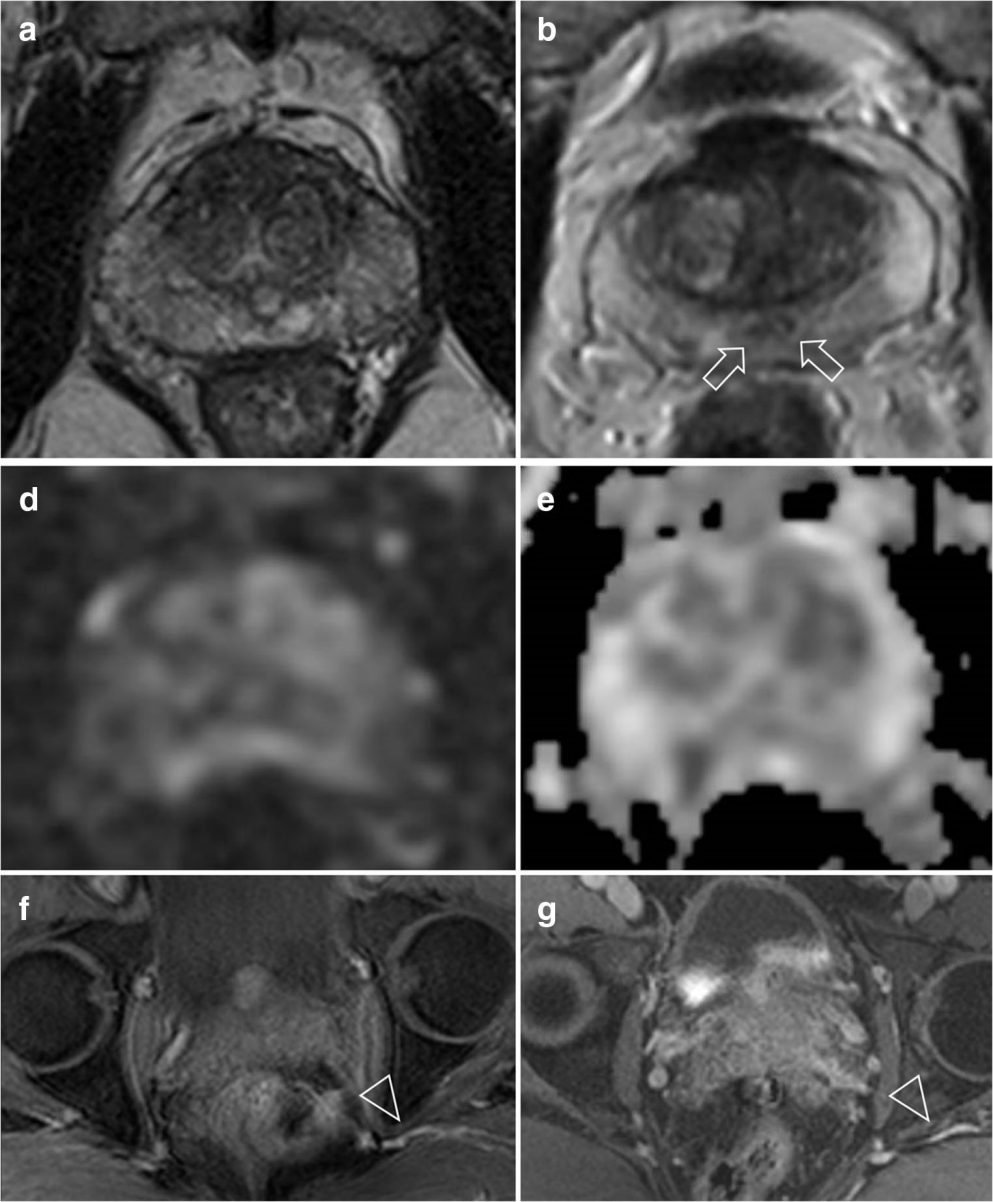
Six examples in which the two readers showed disagreement. *T2-weighted imaging*: in a the disagreement pertained to the delineation of the prostatic capsule while in b of the ejaculatory ducts (arrows). The final consensus was that the two scans were of suboptimal image quality. *Diffusion-weighted imaging*: in d the disagreement pertained to the adequacy of the ADC map (the corresponding high b sequence is provided in c for the sake of completeness). The final consensus was that the ADC map was not of adequate diagnostic quality. *Dynamic-contrast enhanced sequences*: the arrowheads in e and f are indicating the vessels in the Alcock’s (or pudendal) canal in two different patients. One reader scored both scans as of suboptimal quality but after consensus meeting the readers agreed that the Alcock’s (or pudendal) canal was clearly delineated in the two scans
